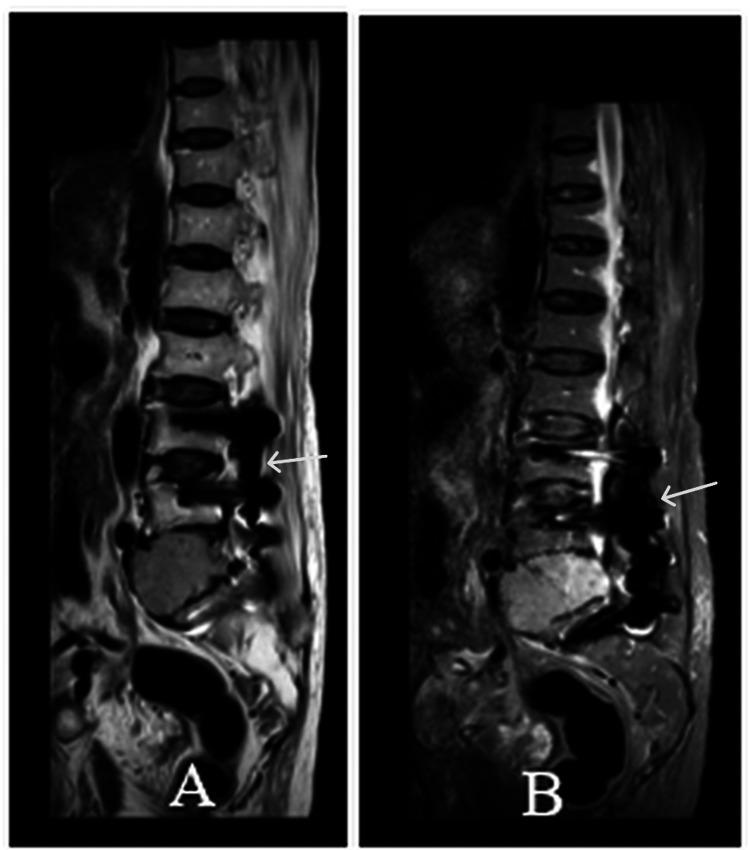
Postoperative MRI (Case 5) (A) Sagittal T2-weighted image: L5 laminectomy changes and posterior spinal fixation extending from L3 to S1 (arrow). (B) Short tau inversion recovery (STIR) sequence: maintained postoperative spinal alignment with reduction in inflammatory soft tissue changes at the operative site (arrow). Note: This figure is presented as a postoperative MRI demonstrating spinal alignment and instrumentation following surgical stabilization. As the corresponding preoperative MRI images were not available for review, direct radiological comparison was not possible. In addition, interpretation may be influenced by metallic susceptibility artifacts related to pedicle screw fixation. Treatment response is described primarily on the basis of clinical improvement and follow-up findings.
